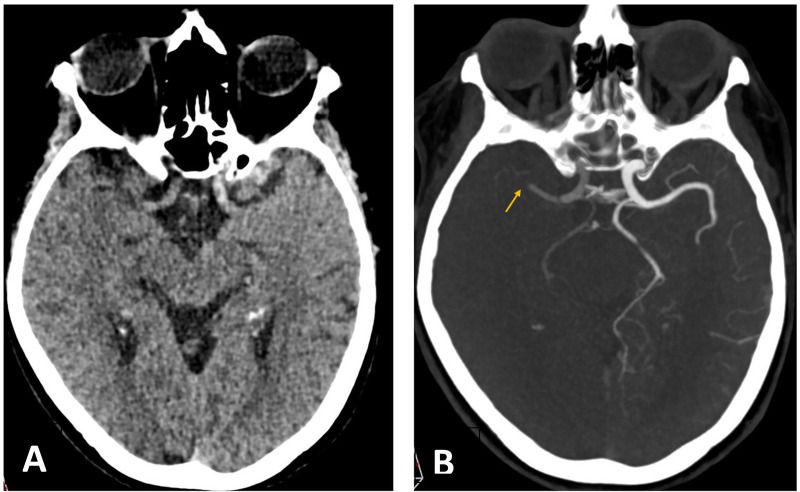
A 76-year-old woman was admitted to hospital with a right hemispheric stroke. Her National Institutes of Health Stroke Scale (NIHSS) score was 18. Non-contrast CT (A) showed no signs of established cerebral infarction. CT angiography (B) revealed an occlusion of the M1 segment of the right middle cerebral artery (MCA) (arrow).
